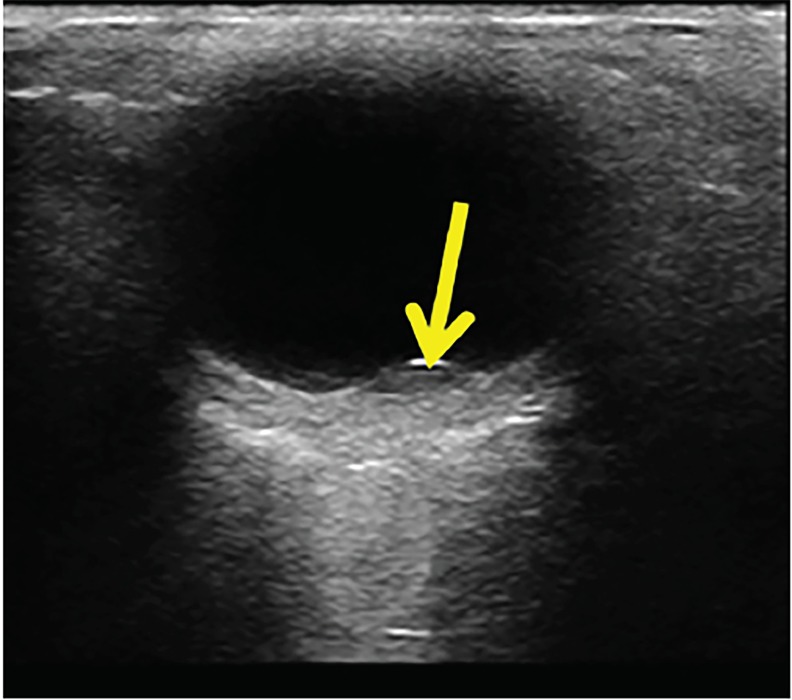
Point-of-care ultrasound demonstrating a pre-retinal hemorrhage (arrow).
